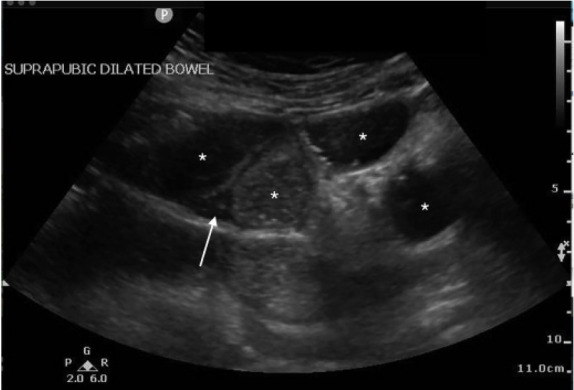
Point-of-care ultrasound demonstrating short-axis view of dilated loops of bowel (*) with localized surrounding free fluid (white arrow) consistent with small bowel obstruction.
